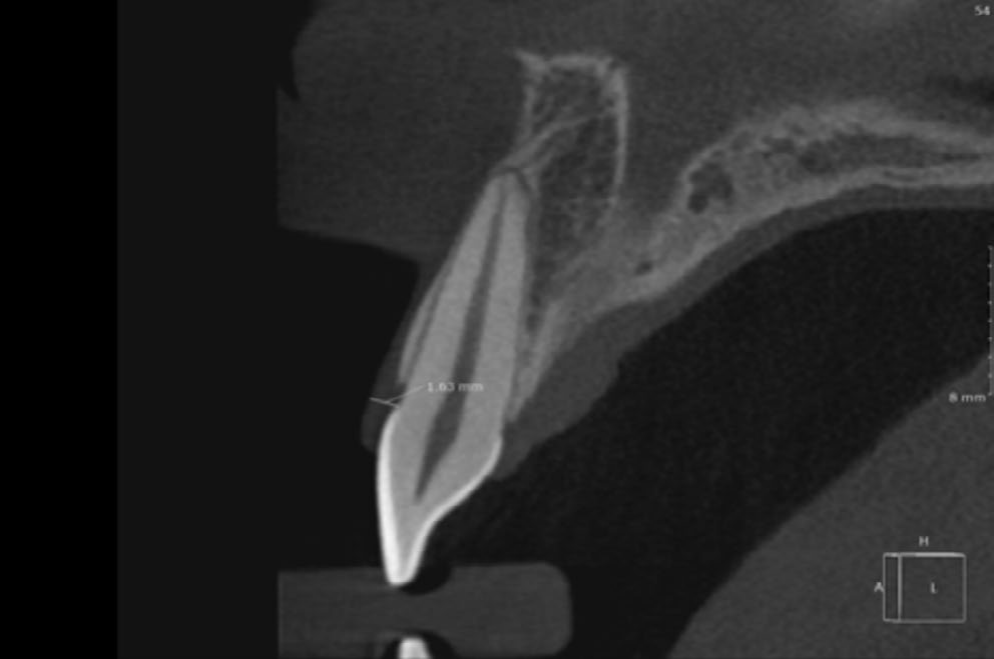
Sagittal section of the cone beam computed tomography, indicating the measurement of gingival thickness perpendicular to the cementoenamel junction.
